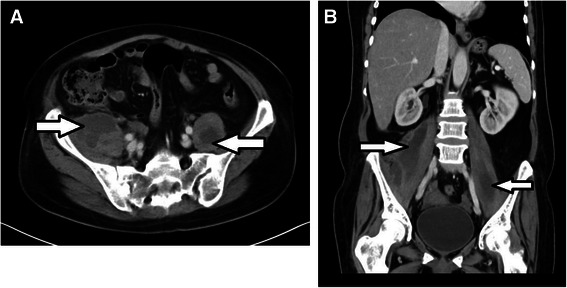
Axial (a) and coronal (b) abdomen and pelvis computed tomography scan with intravenous contrast showing bilateral psoas muscle abscesses (*arrows*)
